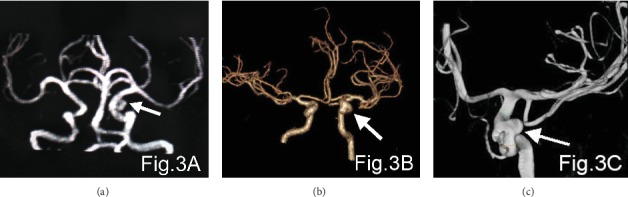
(a–c) show the MRA, CTA, and 3D-DSA, respectively, of the left internal carotid aneurysm in the same patient. The arrows show the aneurysm.
